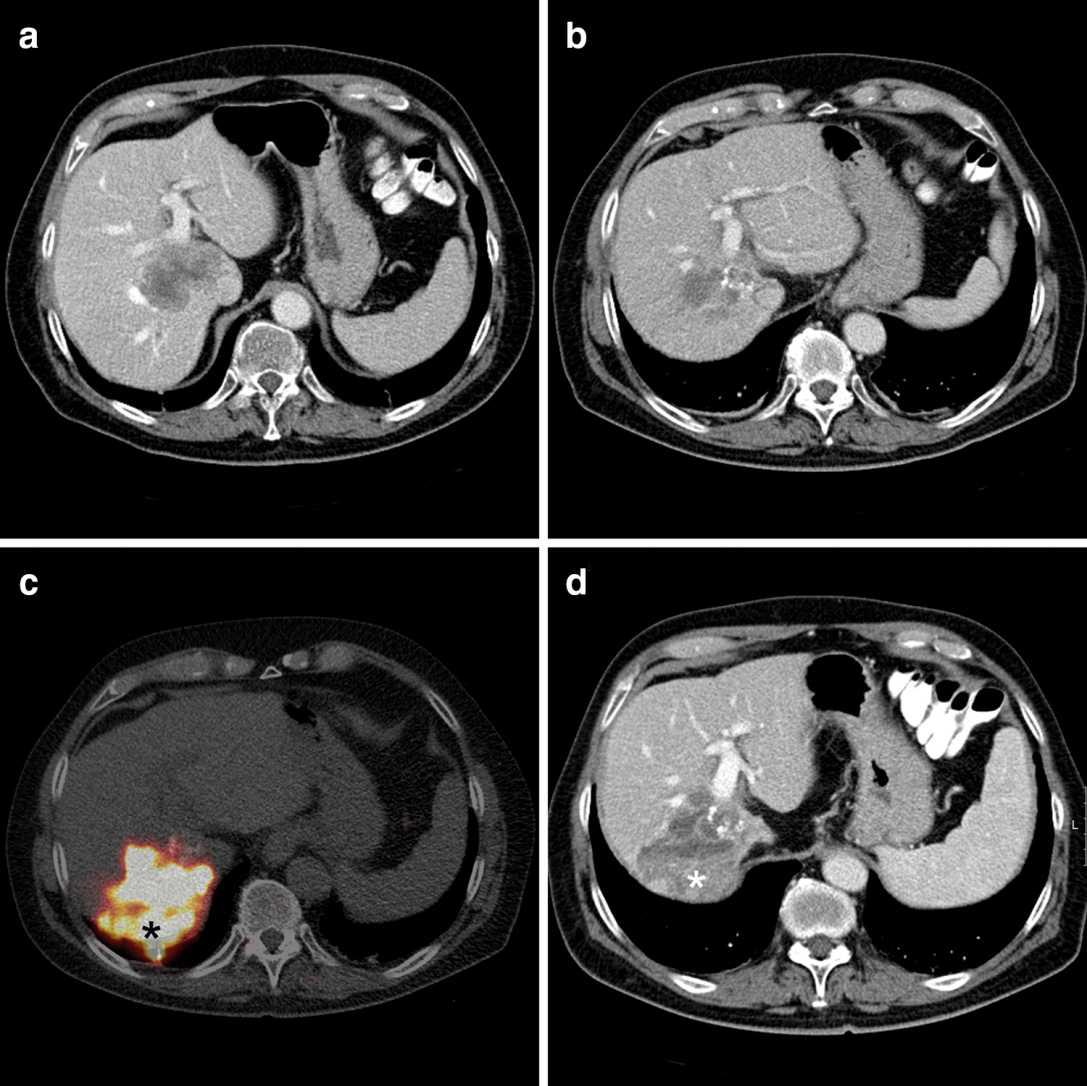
Induction of hypertrophy after 2 RE-treatments in a patient with CRLM. a CT scan prior to the first treatment with a CRLM located centrally in the right hemiliver, also involving the caudate lobe. b Three months after a whole liver treatment a decrease in the lesion size is seen. Segment 2–3 have hypertrophied (degree of hypertrophy: 16 %). c
